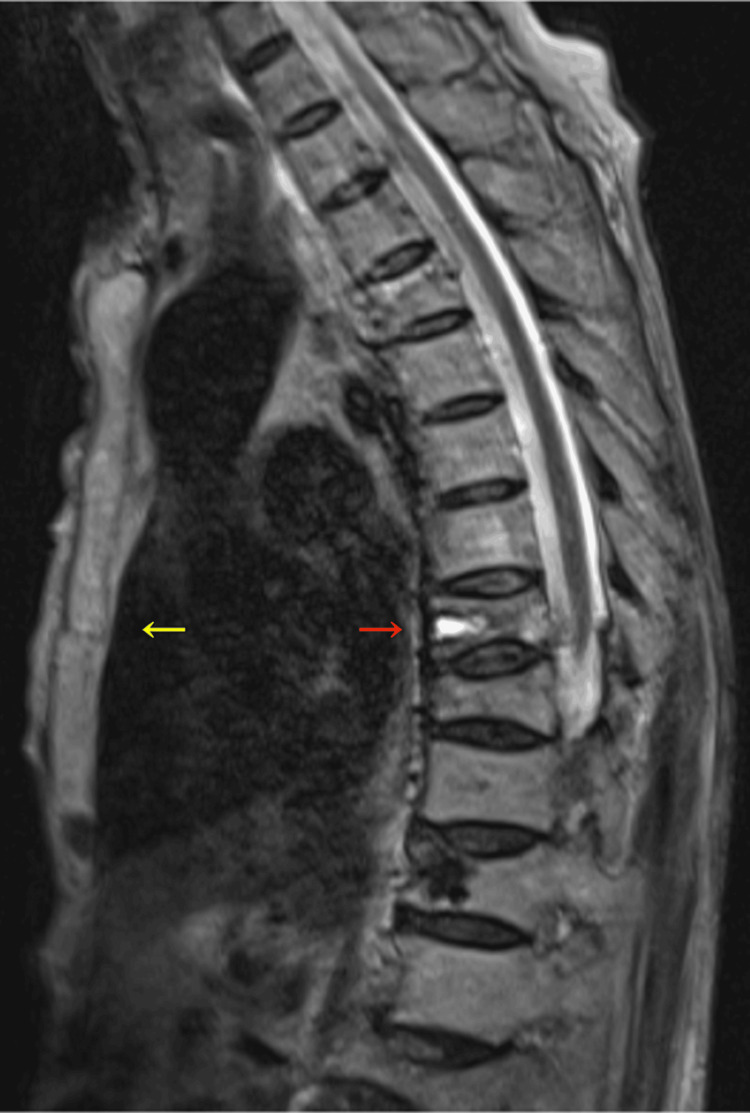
T2-weighted magnetic resonance imaging showing a sternal fracture (yellow arrow) and a thoracic spine fracture (red arrow) at the same segmental level.
